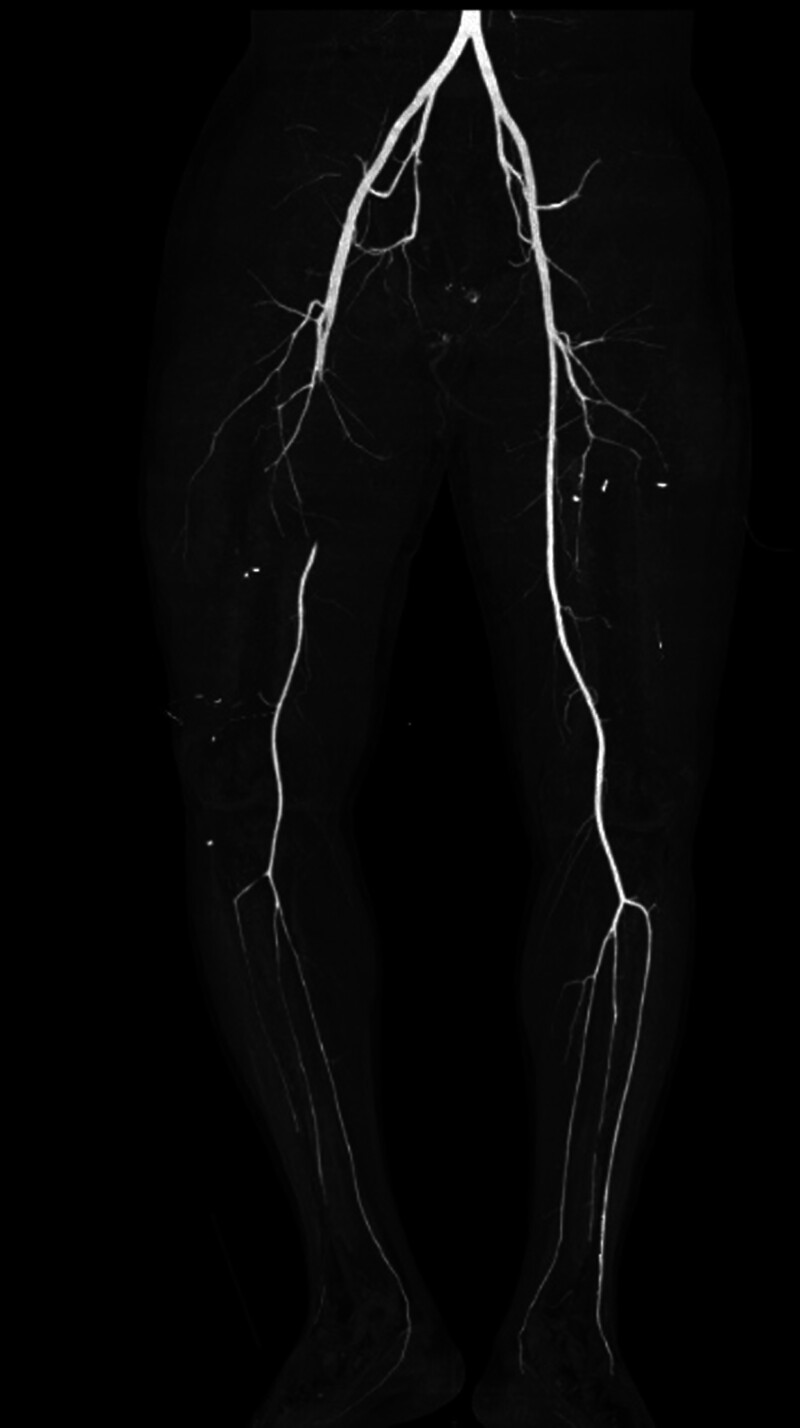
CTA showing a long-segment embolism of the right femoral artery. CTA =
